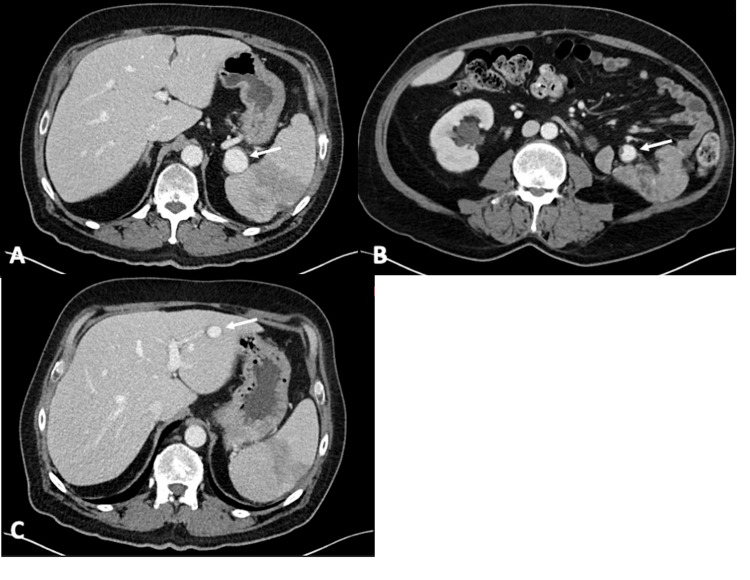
Axial contrast-enhanced CT images demonstrated a 2.7 cm pseudoaneurysm of the splenic artery (A), a 1.6 cm pseudoaneurysm in the small bowel mesentery (B), and a 1.5 cm pseudoaneurysm in the left lobe of the liver (C). Arrows point to the pseudoaneurysms.
